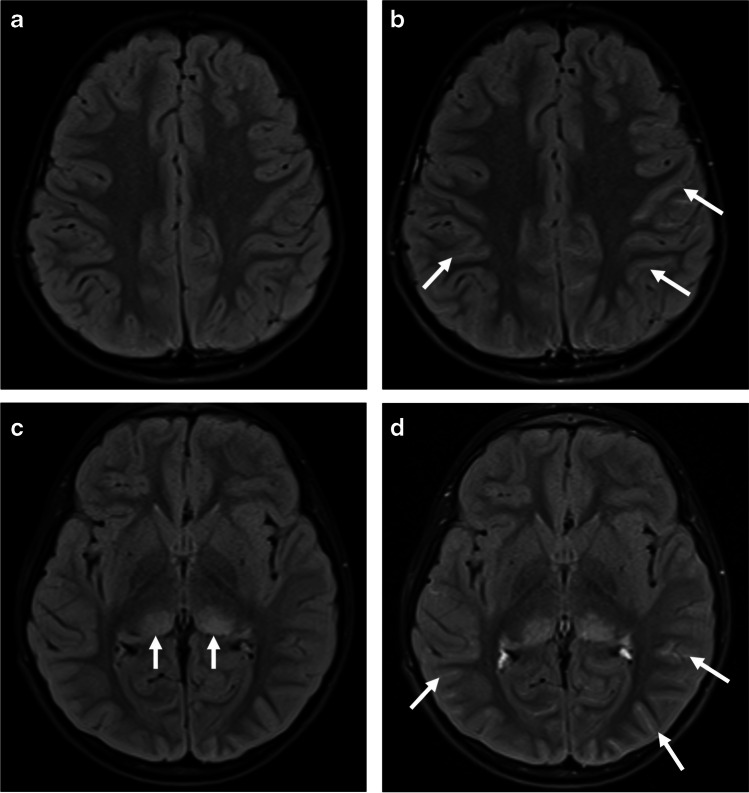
Myelin oligodendrocyte glycoprotein antibody-associated autoimmune encephalitis in a 6-year-old boy, presented with headaches and fever. a, c Axial fat-suppressed fluid-attenuated inversion recovery (FLAIR) and (b, d) axial fat-suppressed contrast-enhanced FLAIR magnetic resonance images show bilateral faint leptomeningeal enhancement, more prominent on the left side compared to the right side (*arrows* in b and d). In addition, there is a symmetric increased signal in the thalami (*arrows* in c), but no enhancement (d)
